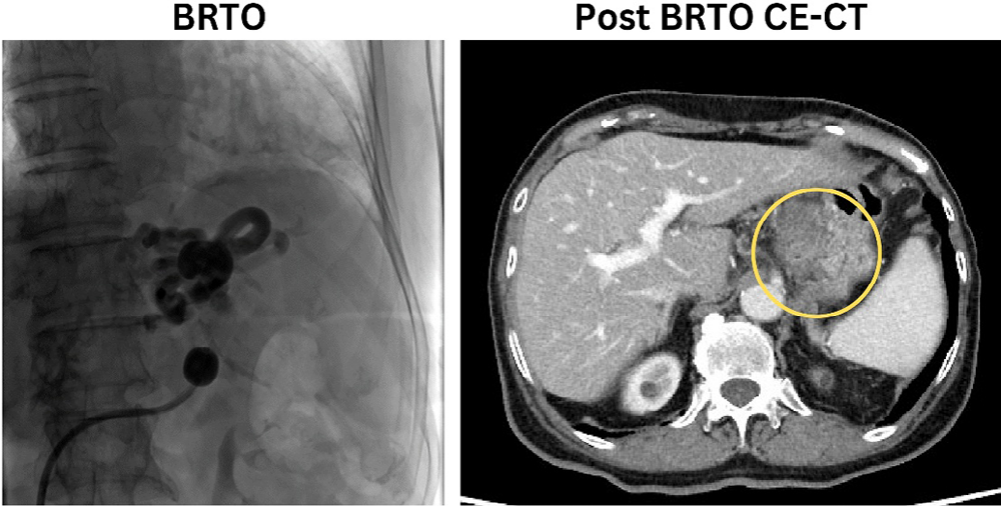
The venogram of balloon‐occluded transfemoral obliteration (BRTO) procedure. Post‐BRTO contrast‐enhanced computed tomography (CE‐CT) reveals occlusion of the portosystemic shunt (circle).
